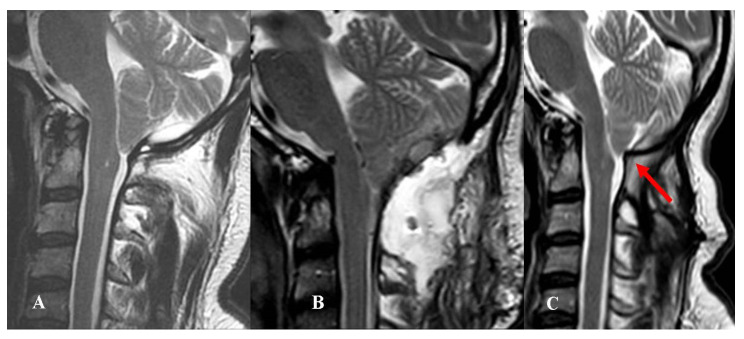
This 48-year-old man complained of an invalidating Valsalva-related headache. (A) Preoperative MRI, T2-weight, sagittal view showing a CM-1.5. Osteodural decompression was performed, and the arachnoid was accidentally violated. The dural sac was repaired using biological graft and fibrin glue. (B) Postoperative MRI, T2-weight, sagittal view showing a deep extradural pseudomeningocele that was not evident at skin level. Conservative treatment was attempted at first. The headache continued to worsen, especially in orthostatism, and 4 months later, he underwent reoperation for duraplasty using autologous fascia lata. (C) Follow-up MRI, T2-weight, sagittal view obtained 9 months after reoperation. There was no evidence of CSF collection; however, the orthostatic pain improved, but the headache did not yet resolve. Adhesions may have developed between the autologous graft and the cerebellum (red arrow). A further surgical procedure was offered, but was refused by the patient. The final CCOS score was 12.
